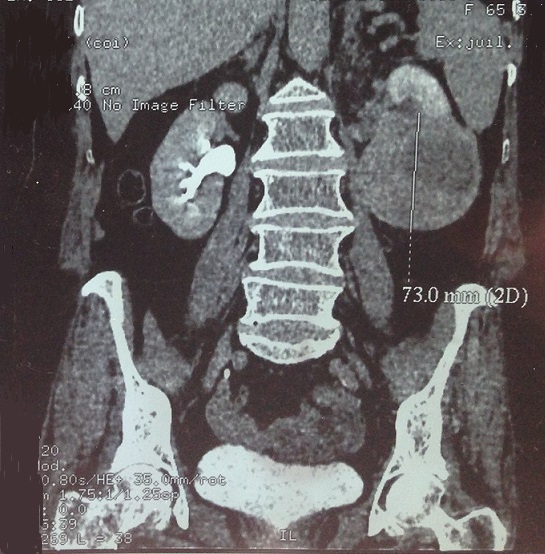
Abdominal and pelvic computed tomography scan showed the presence of a large tissue process at the upper pole of the right kidney measuring 8 cm of diameter, demonstrating heterogeneous contrast enhancement
